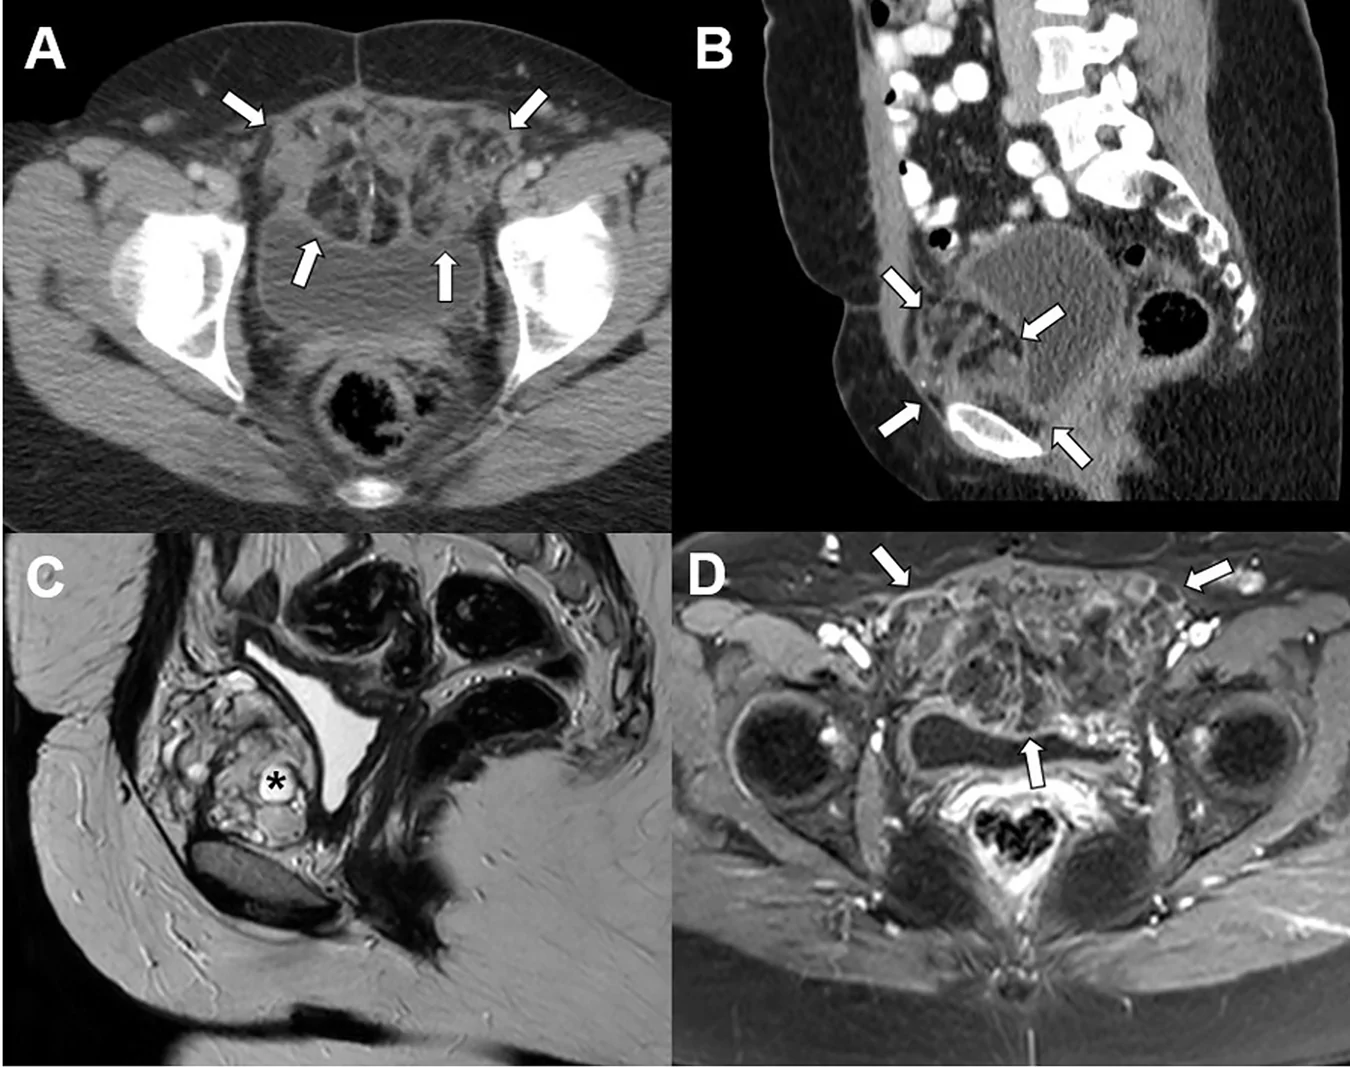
Pathologically proven malignant immature teratoma in the space of Retzius. Axial and sagittal (A, B) CT images show a lobulated macroscopic fat-containing mass in the space of Retzius (arrows). C Sagittal T2-weighted MR image demonstrates cystic areas within the mass (asterisk). D Axial post-contrast T1-weighted MR image shows heterogeneous enhancement of the lesion
